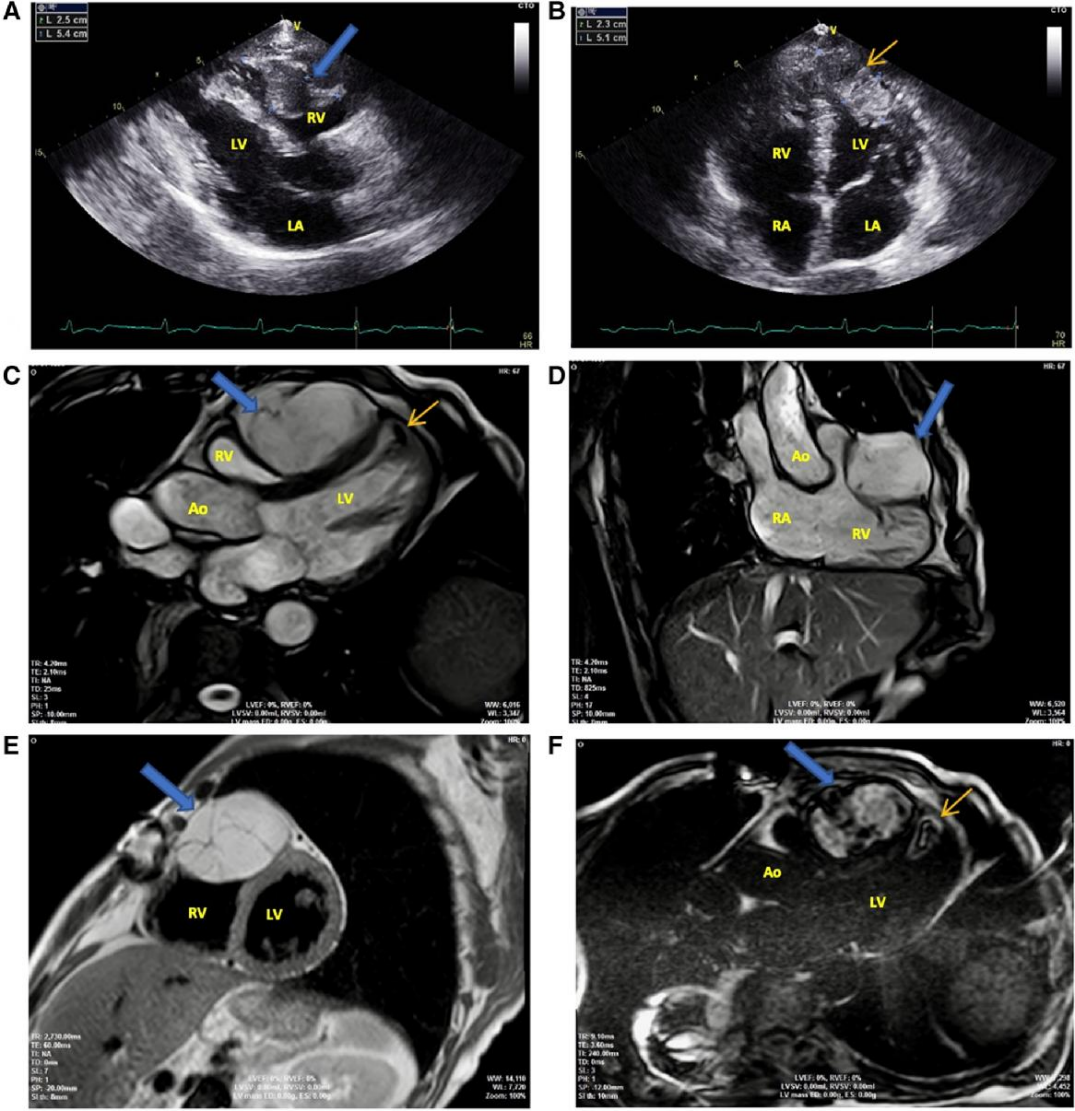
Transthoracic echocardiogram (TTE) and cardiac magnetic resonance imaging (CMR) findings. (*A*) TTE parasternal long-axis view revealing an oblong, non-vascularized intra-myocardial mass attached to the right ventricle (RV) free wall’s myocardium (blue arrow). (*B*) TTE apical view revealing an oblong non-vascularized intra-myocardial mass in the antero-septal-apical wall of the left ventricle (LV), extending to the antero-lateral-apical wall (orange arrow). (*C*) CMR three-chamber view showing an intra-myocardial mass on the anterior wall of the RV (blue arrow) with compression effect associated with a small mass on the antero-septal-apical wall of the LV (orange arrow). (*D*) CMR RV outflow view showing an intra-myocardial mass on the anterior wall of the RV (blue arrow). (*E*) Fat–water separation sequences in short-axis view characterizing the non-adipose nature of the RV mass which appears in higher signal intensity with the presence of septations. (*F*) Late gadolinium enhancement (LGE) sequences in three-chamber view showing a heterogeneous signal of the two cardiac masses.
